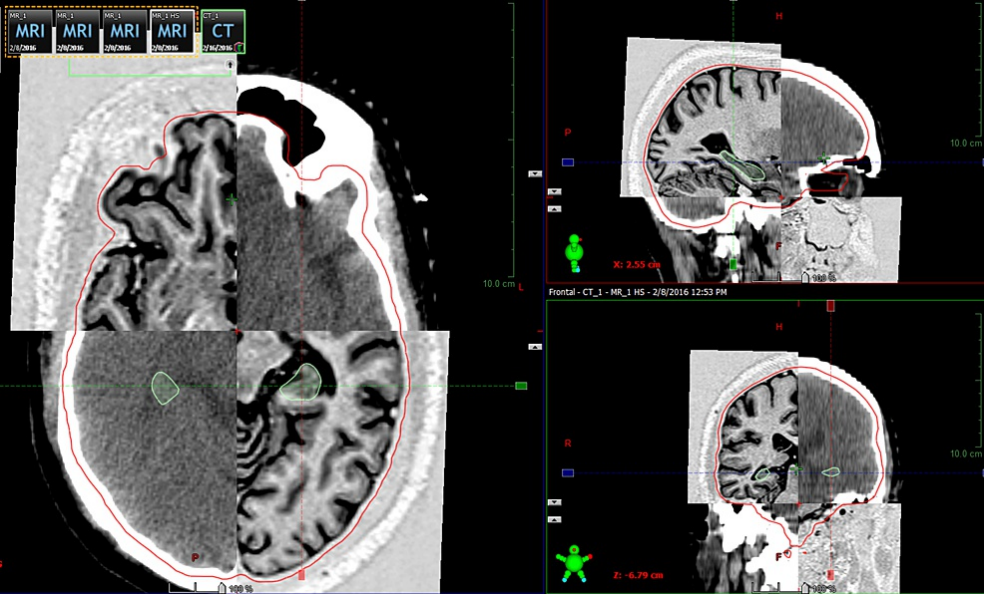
Hippocampus contouring using magnetic resonance imaging (MRI) registration. Three-dimensional image showing contouring of the hippocampus in green color.
